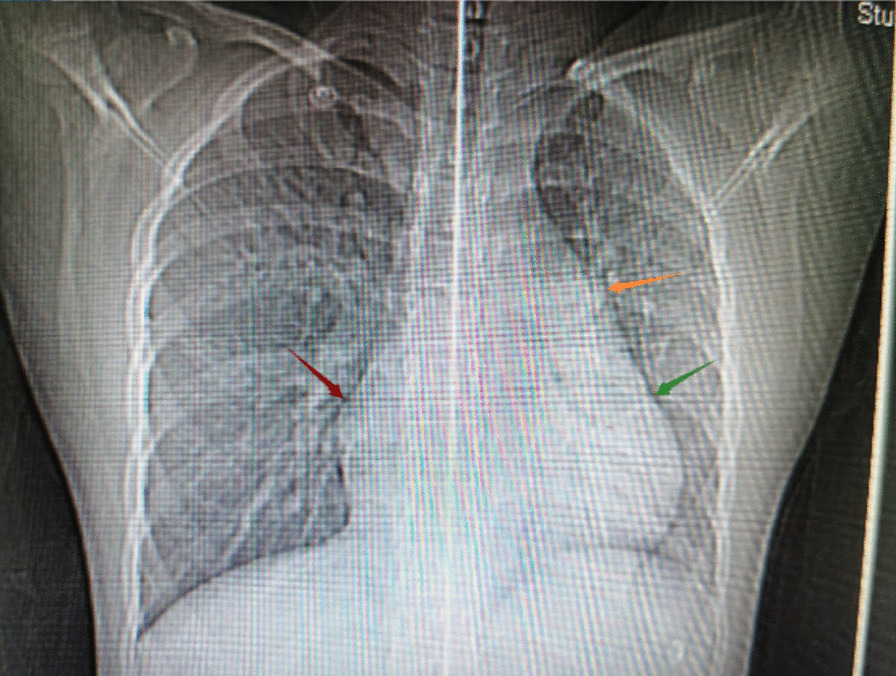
Chest X-ray of the patient. The yellow arrow represents the widening of the pulmonary artery segment; the red arrow represents the enlargement of the RA; and the green arrow represents the enlargement of the RV
